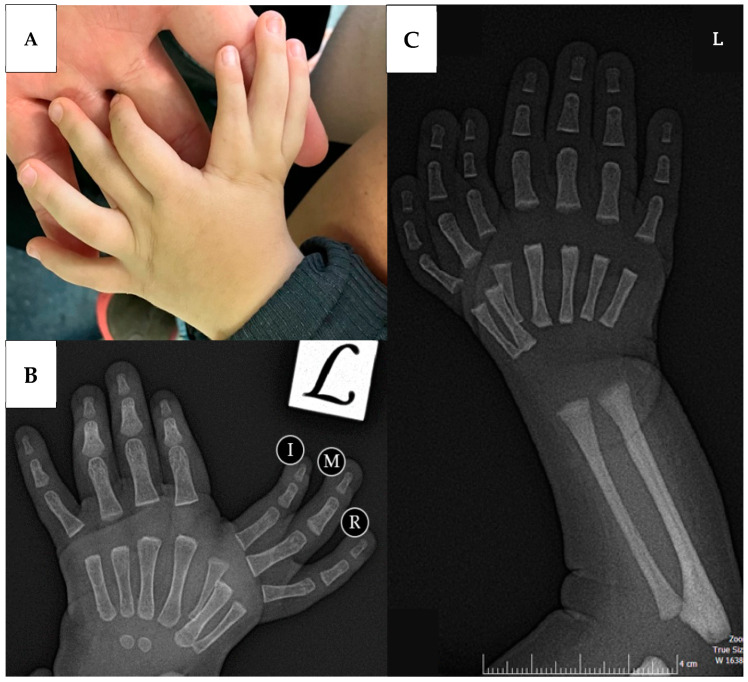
An image of the child’s left hand during the physical examination, where four postaxial and three ulnarly deviated preaxial triphalangeal fingers are visible (A). The pre-operative X-ray in the antero-posterior (AP) position of the patient’s left hand demonstrating a hypoplastic metacarpus of I and well-developed M and R fingers (B). The pre-operative (AP) radiograph of the child’s left forearm showing a properly formed ulna and radius (C).
